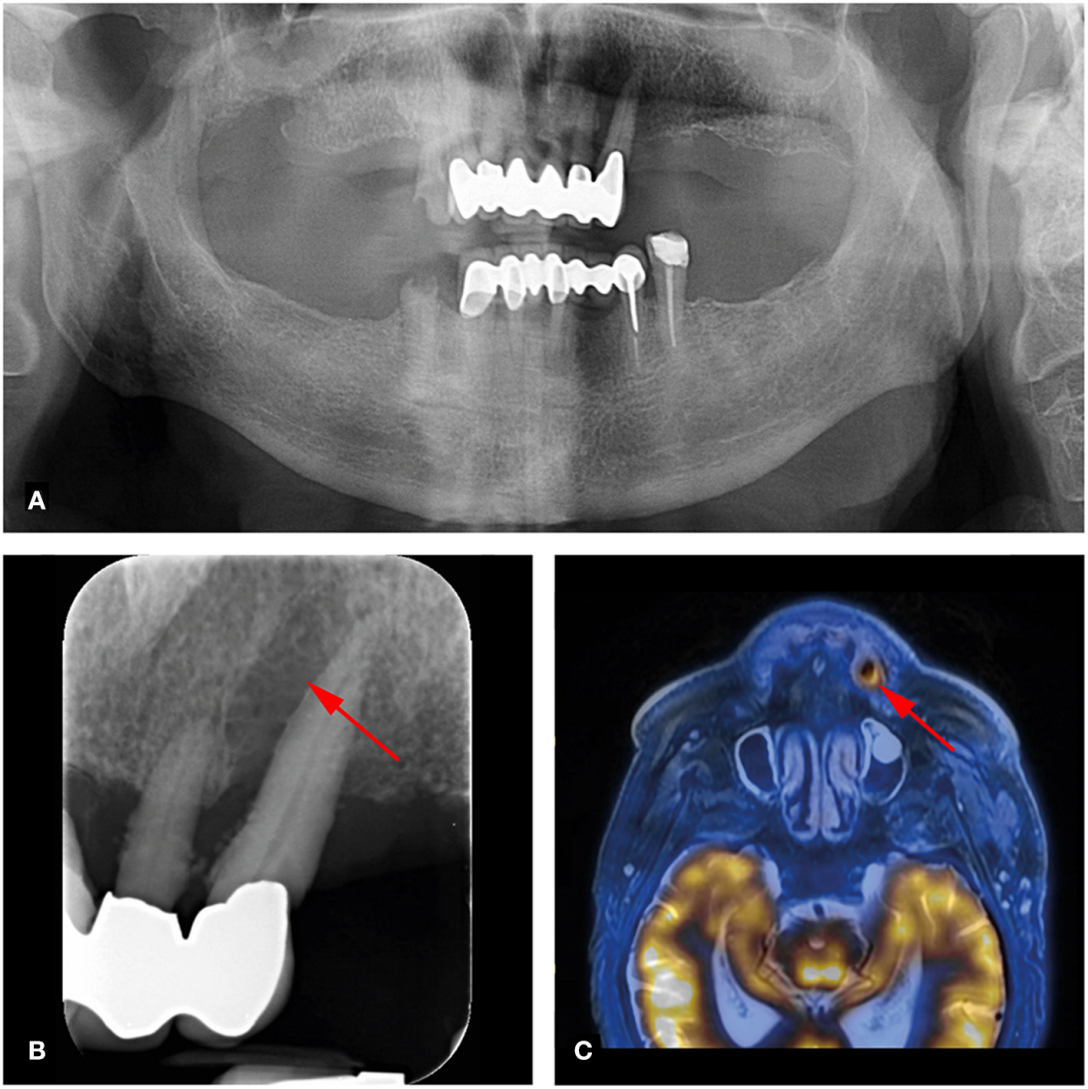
73-year-old man with right-sided hypopharynx carcinoma cT3 cN2b cM0. Panoramic (A) and dental radiography (B) shows a periapical lesion with marginal bone loss in region 23 (arrow; PAI score 4, MPI score: 4). (C) FDG-PET/MR shows a metabolically active osteolysis at tooth 23 with a SUVmax of 6.8 (arrow).
